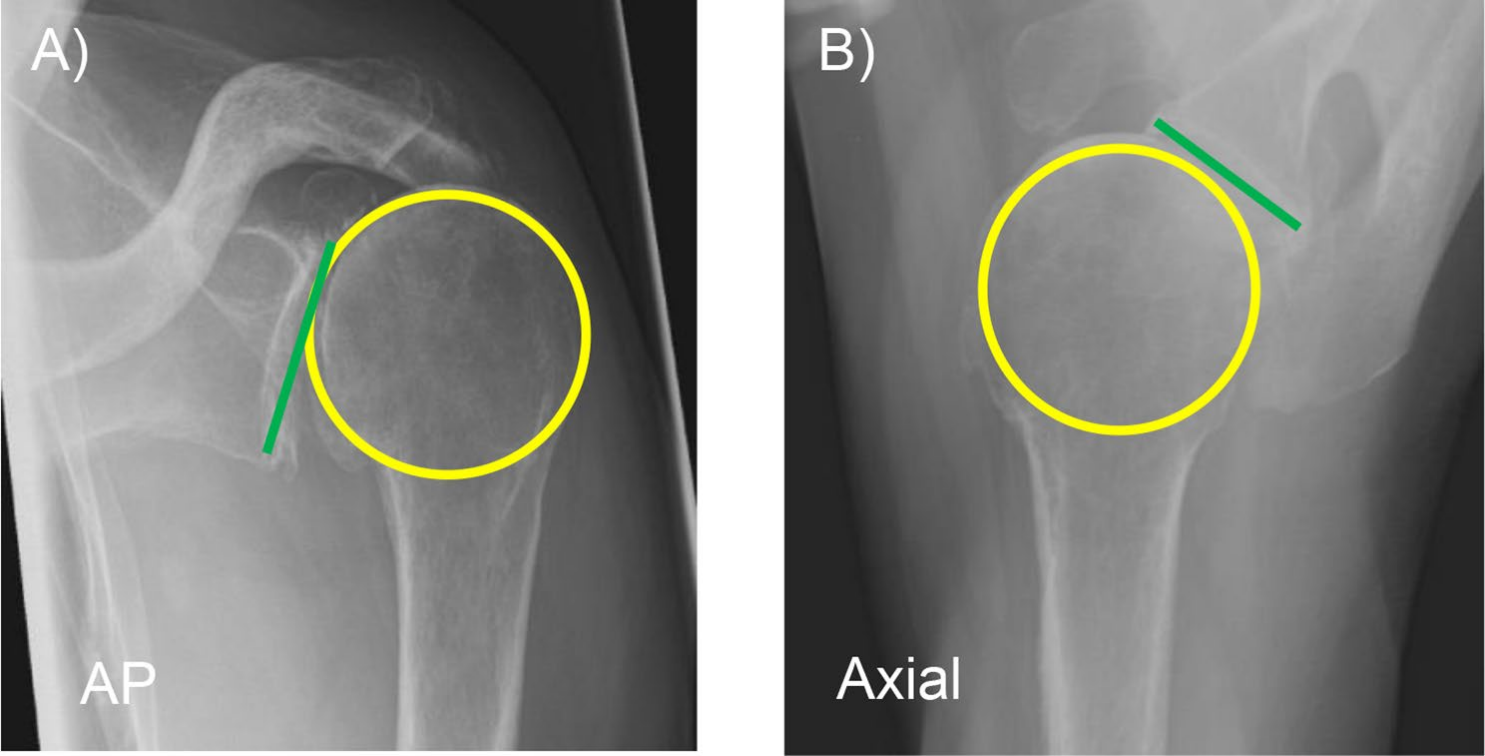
Radiologically determination of best-fit circle of the humeral head in yellow. The humerus height and width appears colored in green in anteroposterior (AP) (A) and axial (B) radiographs
